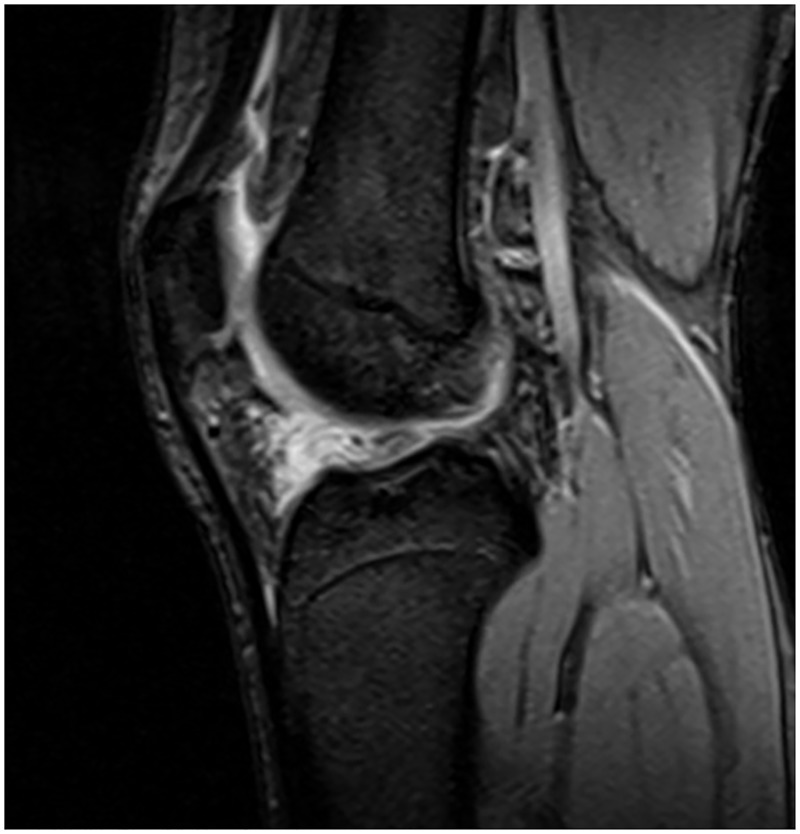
Sagittal proton density-weighted MRI of the knee of a 21-year-old male show that the disorder and irregular strip-like signal was observed in the AHLM and articular capsule.
